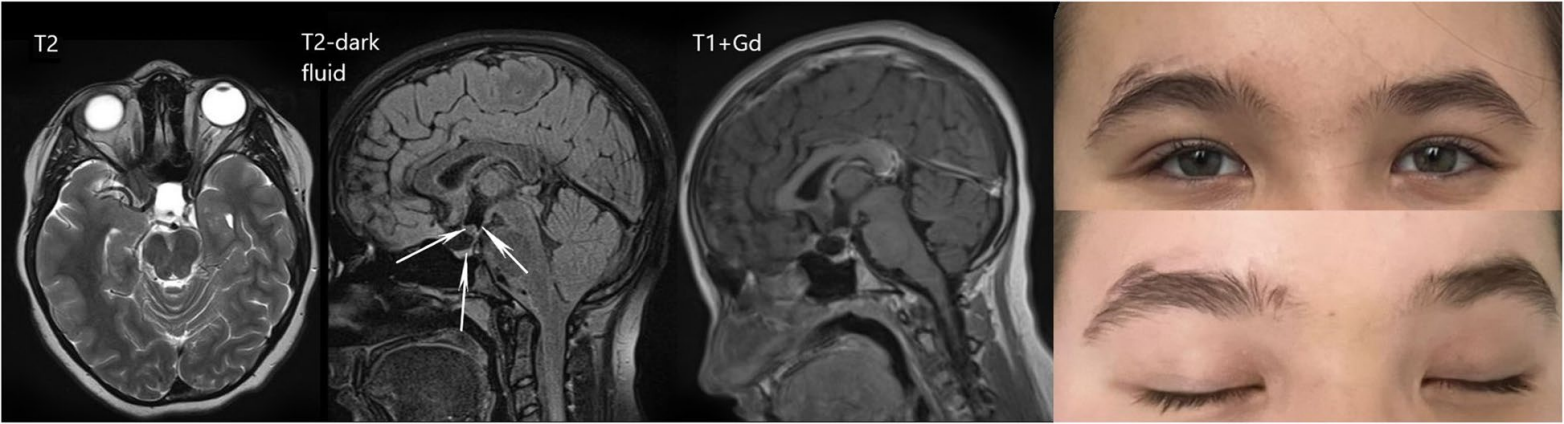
Follow-up in 6 months: MRI and photos of patient. The 3rd ventricle floor, pituitary gland, and optic chiasm are marked by arrows; cosmetic outcome (left eyebrow)
